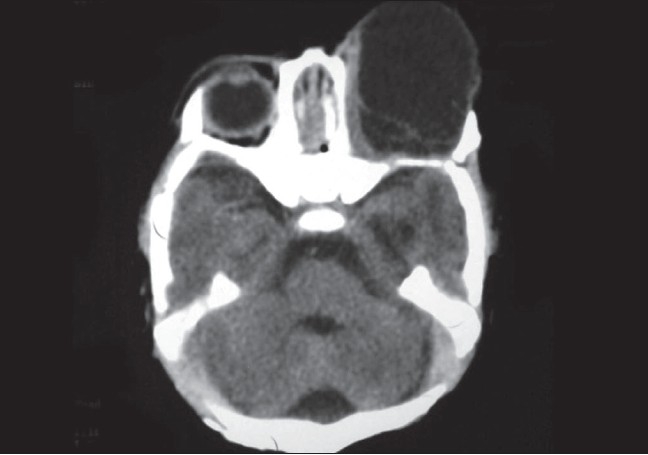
Axial CT scan of orbit showing hypodense cystic area on left side with anophthalmia
